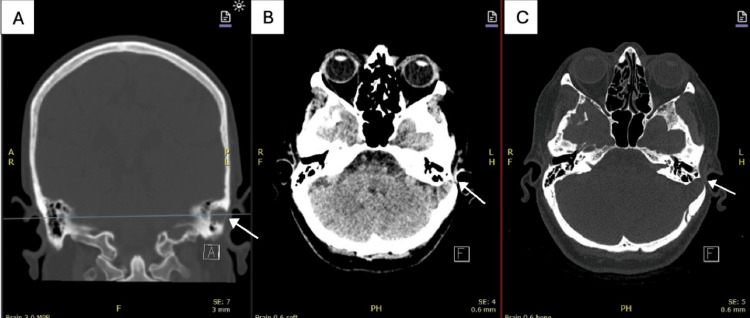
High-resolution computed tomography of the temporal bones. (A) Coronal reconstruction in bone window revealing erosion of the left petrous bone above the labyrinth, confirming tegmen dehiscence with associated cortical thinning with defect and opacification of the mastoid air cells (white arrow). (B) Axial reconstruction in soft tissue window demonstrating a focal bony defect of the left tegmen tympani with soft tissue density herniating into the mastoid air cells (white arrow), consistent with encephalocele and CSF otorrhea. (C) Axial reconstruction in bone window demonstrating a focal bony defect of the left tegmen tympani with soft tissue density herniating into the mastoid air cells (white arrow), consistent with encephalocele and CSF otorrhea.
